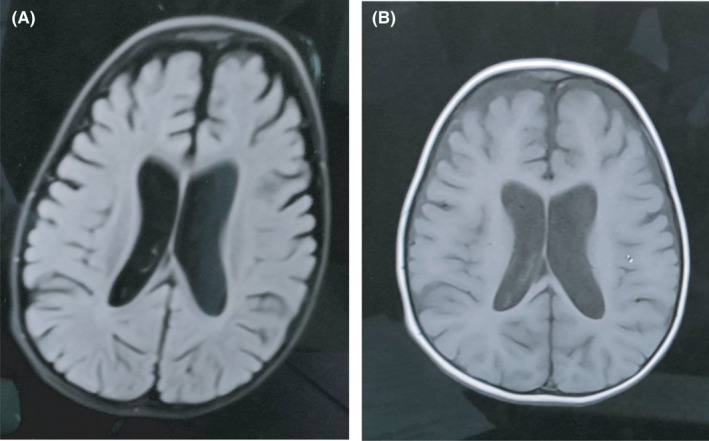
(A) FLAIR (B) T1 weighted showing proportionate dilatation of the ventricles and moderate cerebral atrophy
